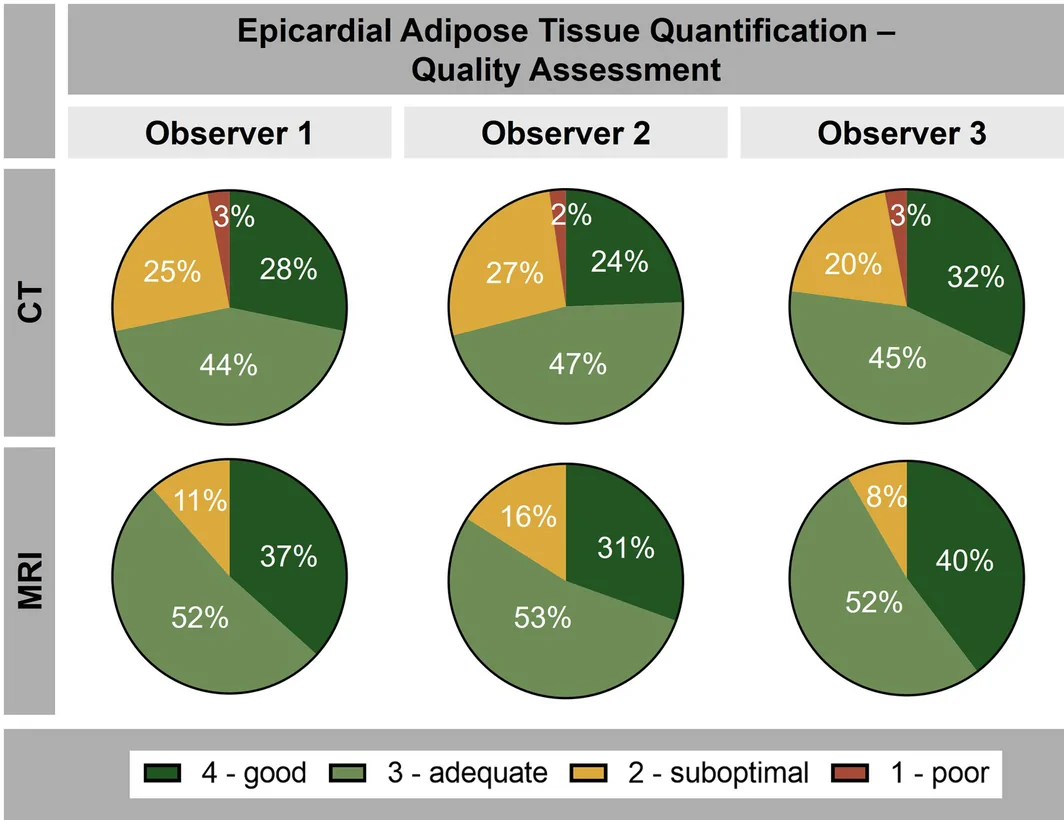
Comparison of image quality for cardiac computed tomography (CT)‐based and magnetic resonance imaging (MRI)‐based epicardial adipose tissue quantification.
